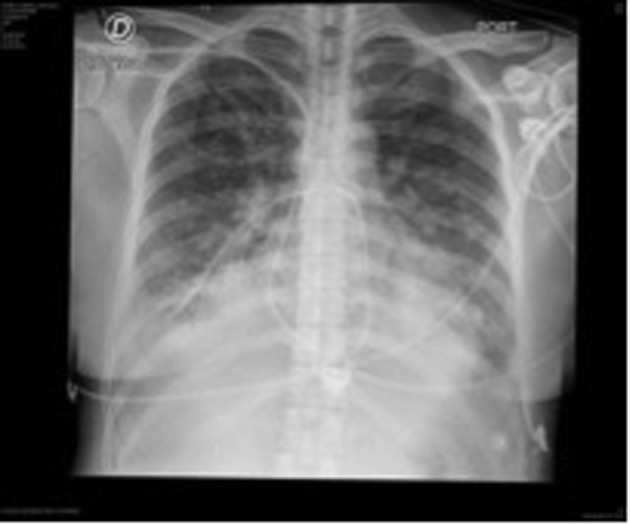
Following thoracic X-rays showing signs of pulmonary edema.
